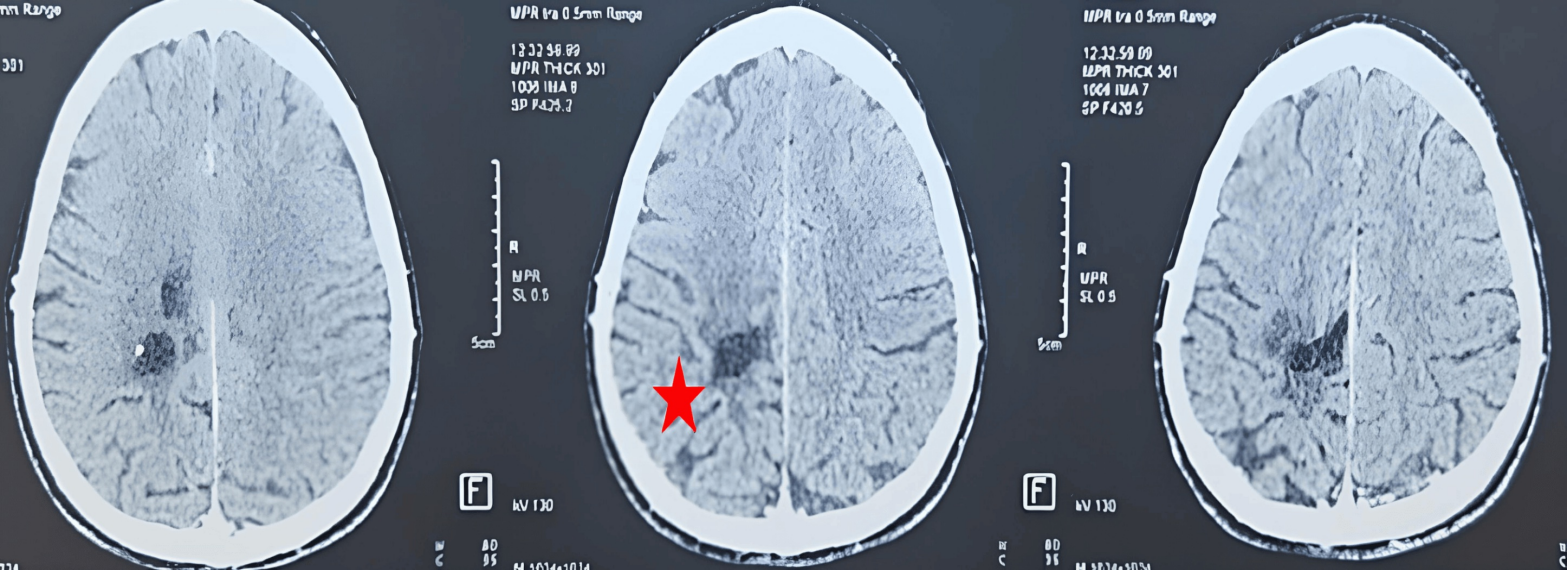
Two-year follow-up brain CT scan. Axial images demonstrate a stable hypodense lesion in the left frontal lobe (red star), without evidence of progression or new contrast enhancement. The overall radiological aspect remains unchanged compared with previous examinations, confirming the long-term stability of the lesion.
